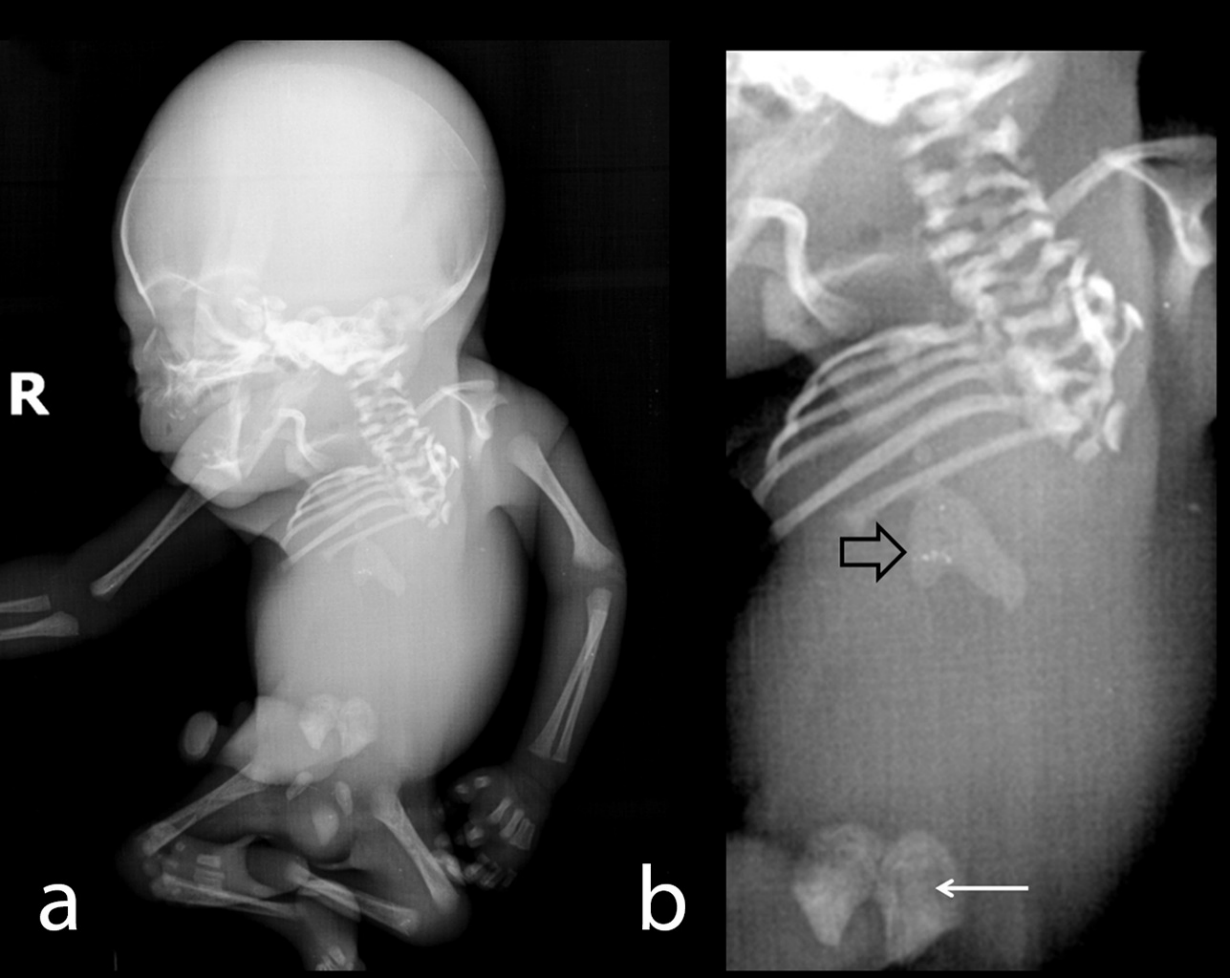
(a) Radiograph of the aborted foetus shows normal skull with the multiple spinal anomalies in cervical and dorsal region. Spinal structures show abrupt termination at the D7 vertebra. Left sided the ribs are absent. Both knee joints are flexed. Long bones of the upper and lower limbs appear normal (b) Close-up view demonstrates spinal anomalies and abrupt spinal termination. Lower sternum-xiphiod bony components shows hypertrophy and a bifid configuration (open arrow). Pelvic configuration is shown in the close-up view (arrow).
